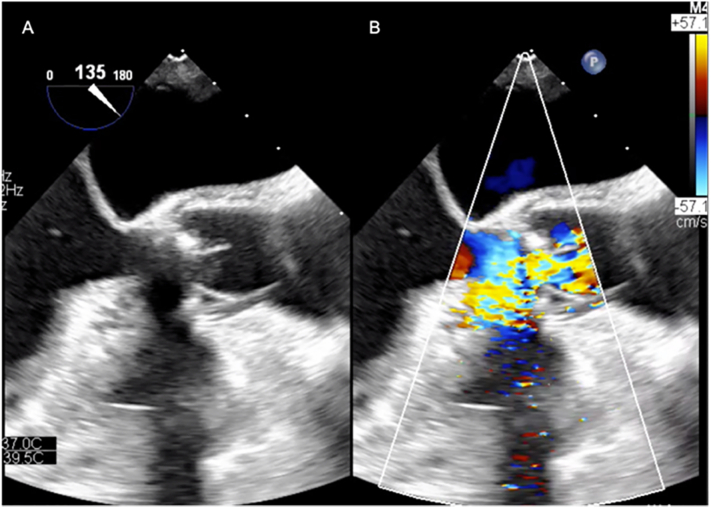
Transesophageal echocardiography. Long axis view; A: diastolic phase, B: diastolic phase with color doppler.
